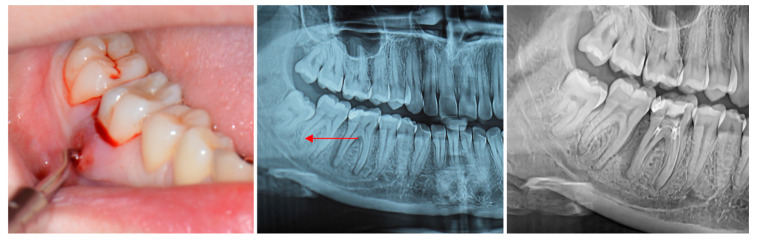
Clinical and radiographic findings of an endo-periodontal lesion of a lower first molar. The intraoral photograph (left) demonstrates localized gingival inflammation and bleeding upon probing in the molar region. The periapical radiograph (center) reveals baseline vertical alveolar bone loss adjacent to the affected tooth (indicated by the red arrow). The third radiographic view (right) shows advanced periodontal bone destruction post-treatment, evidence of failed conventional treatment.
